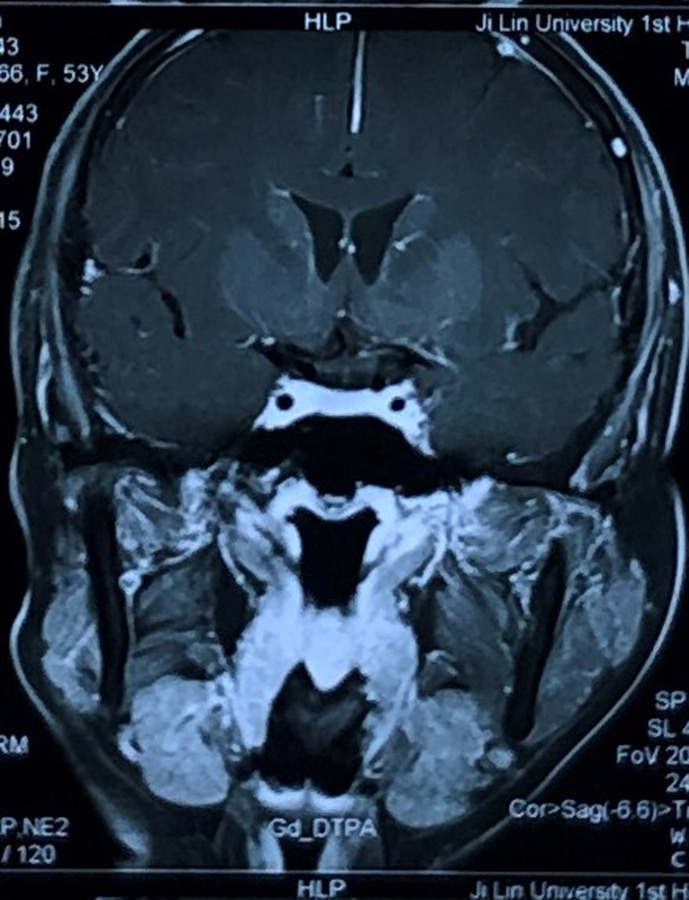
MRI displaying a hypointense lesion
